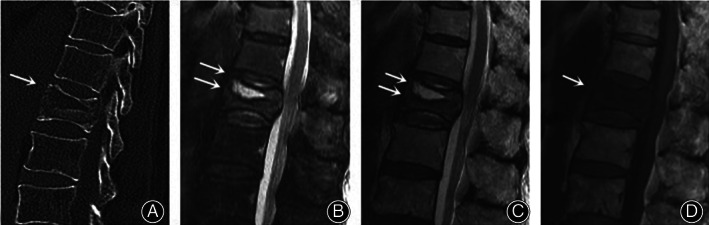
(A) CT image of a 78‐year‐old man showing an IVC in L1. (B) Sagittal STIR image and (C) Sagittal T2‐weighted MRI showing a high linear signal with surrounding low intensity is visible (“double line sign”; arrows). (D) Sagittal T1‐weighted MRI showing a low signal in L1.
